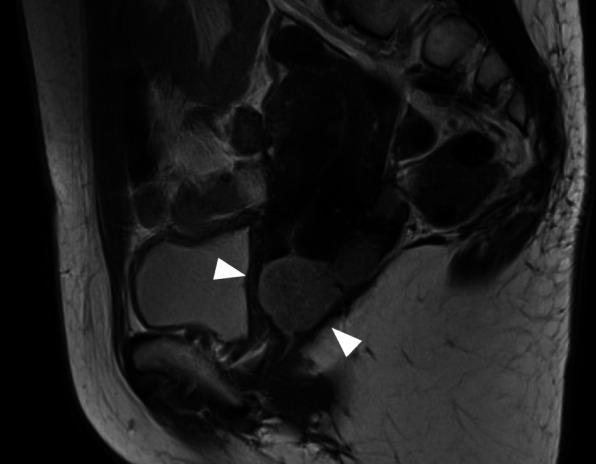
Magnetic resonance imaging of the patient at the initial presentation. A solid tumor developing from the posterior of the uterine cervix was confirmed (arrowheads)
